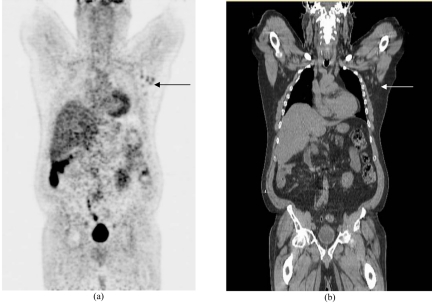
FDG PET-CT scan of the patient. Arrow shows small lymph nodes in the left axilla with the highest standardised uptake value of 3.8 and measuring less than 1 cm in size.
